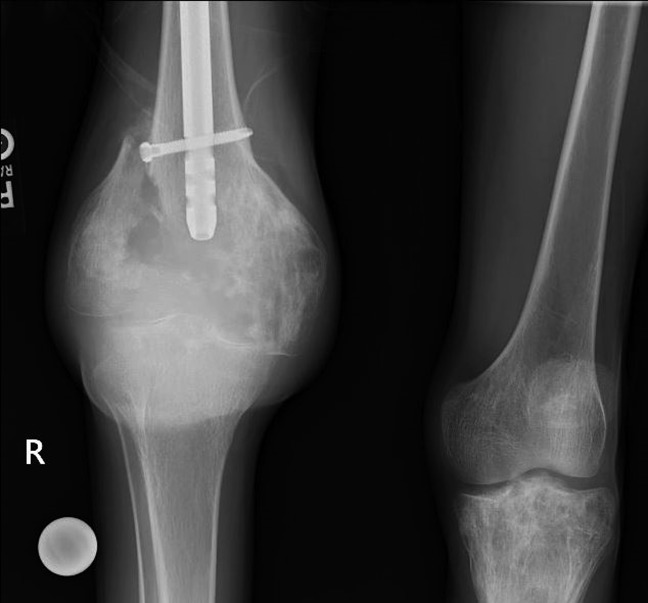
A radiograph 1 month before presentation demonstrating a pathologic fracture of the distal right femur at the apex of the intramedullary nail and extending to the biopsy-proven brown tumor. Left proximal tibia lesion with features of an additional brown tumor.
